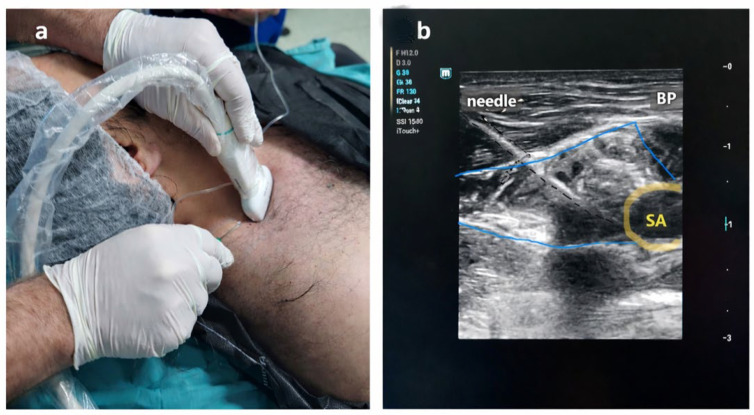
(a,b). Peripheral nerve block (PNB) application. Performing PNB technique. (a) Probe and needle positioning. (b) Ultrasound (US) image. The subclavian artery is indicated by a yellow circular marker, and the brachial plexus is outlined with a blue line. The needle trajectory is represented by a black dashed line. PNB, peripheral nerve block; SA, subclavian artery.
